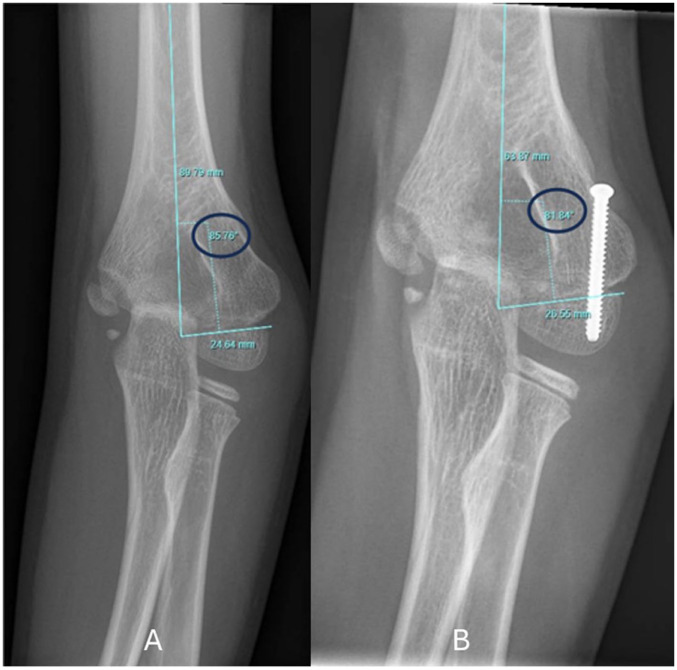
Guided growth around the elbow. Radiographs preoperative (a) and after 1-year follow-up (b). B shows the Baumann angle has improved (circle).
